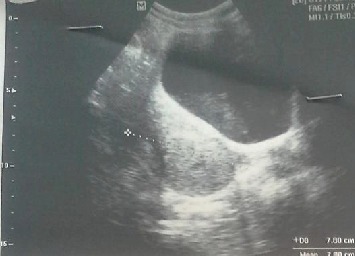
Ultrasonography of uterine inversion.
